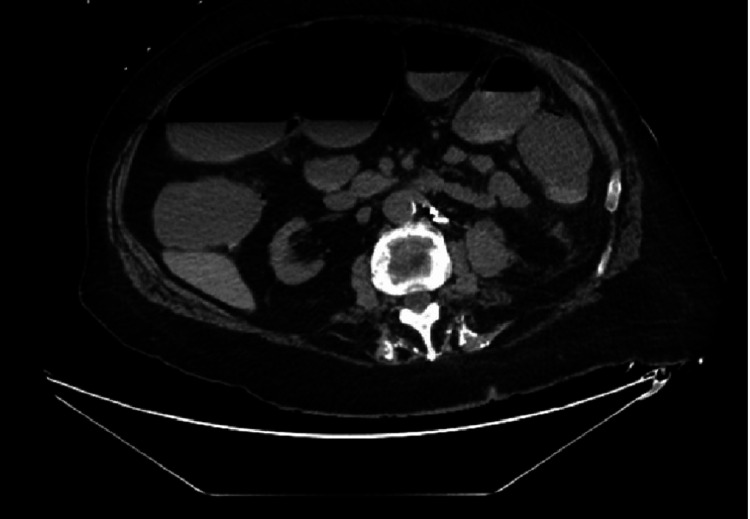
CT angiogram revealed 8 cm constricting lobular soft tissue with high-grade obstruction of the proximal large bowel.
